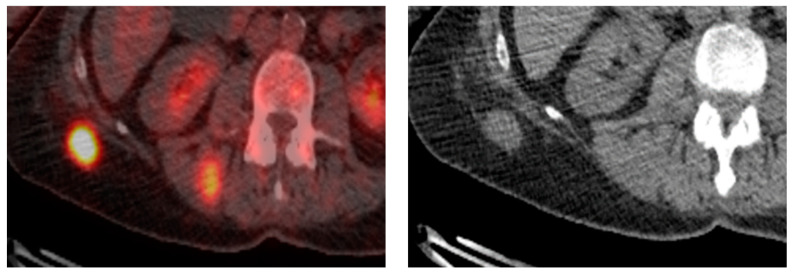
Biopsy-proven Soft tissue EP, case from our institution. Axial non-contrast CT and PET-CT show a well-defined, PET-avid soft tissue nodular lesion in the subcutaneous tissue.
